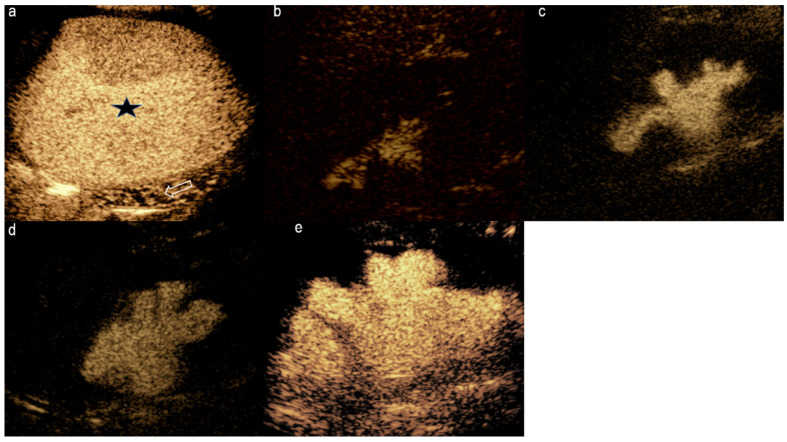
Contrast-enhanced urosonographic staging of vesicoureteric reflux. Images acquired from different children with varying degrees of reflux. (a) Grade I reflux: Contrast limited to the distal ureter. (b) Grade II reflux: Contrast extending to a non-dilated pelvicalyceal system. (c) Grade III reflux: Contrast seen in mildly dilated pelvicalyceal system with preserved calyceal contour. (d) Grade IV reflux: Contrast in moderately dilated pelvicalyceal system with blunted fornices but preserved papillary impression. (e) Grade V reflux: Contrast seen in severely dilated collecting system with fornices blunted and lost papillary impression. Black star: contrast filled bladder. White arrow: distal ureter.
